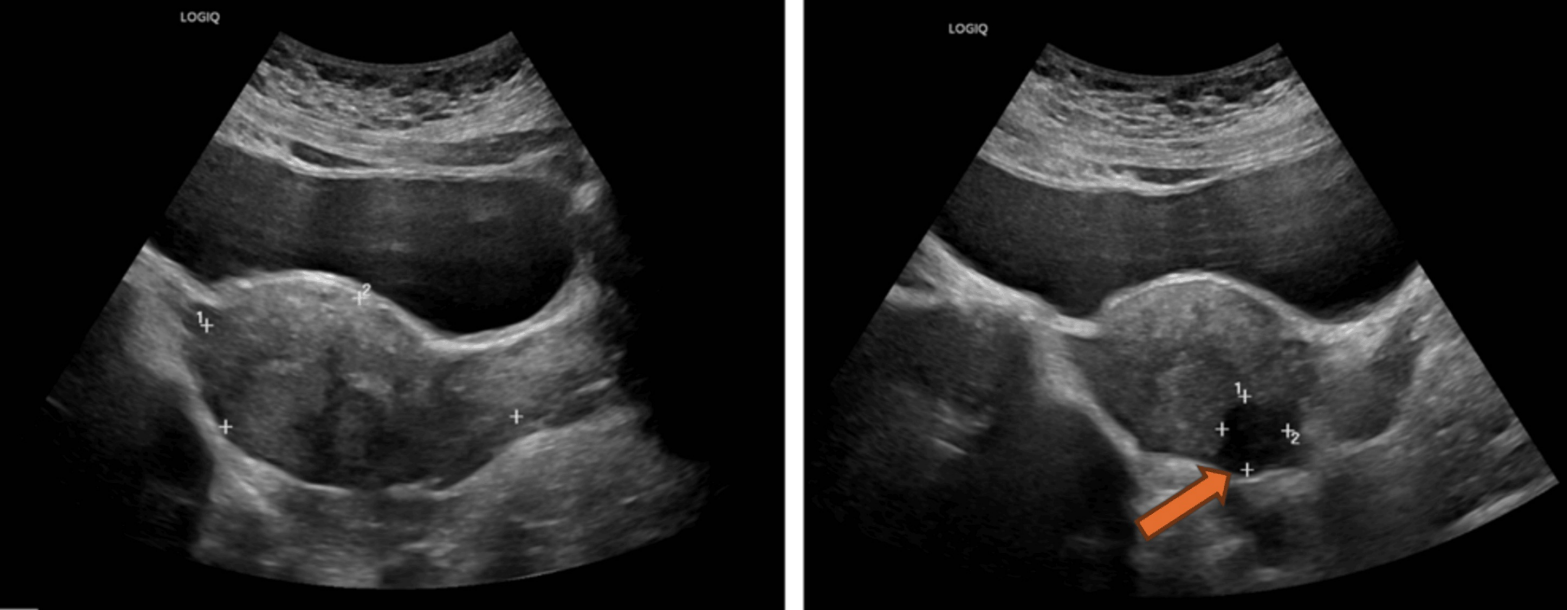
Pelvic ultrasound showing a small posterior wall intramural uterine fibroid measuring 1.9 × 1.7 cm.
